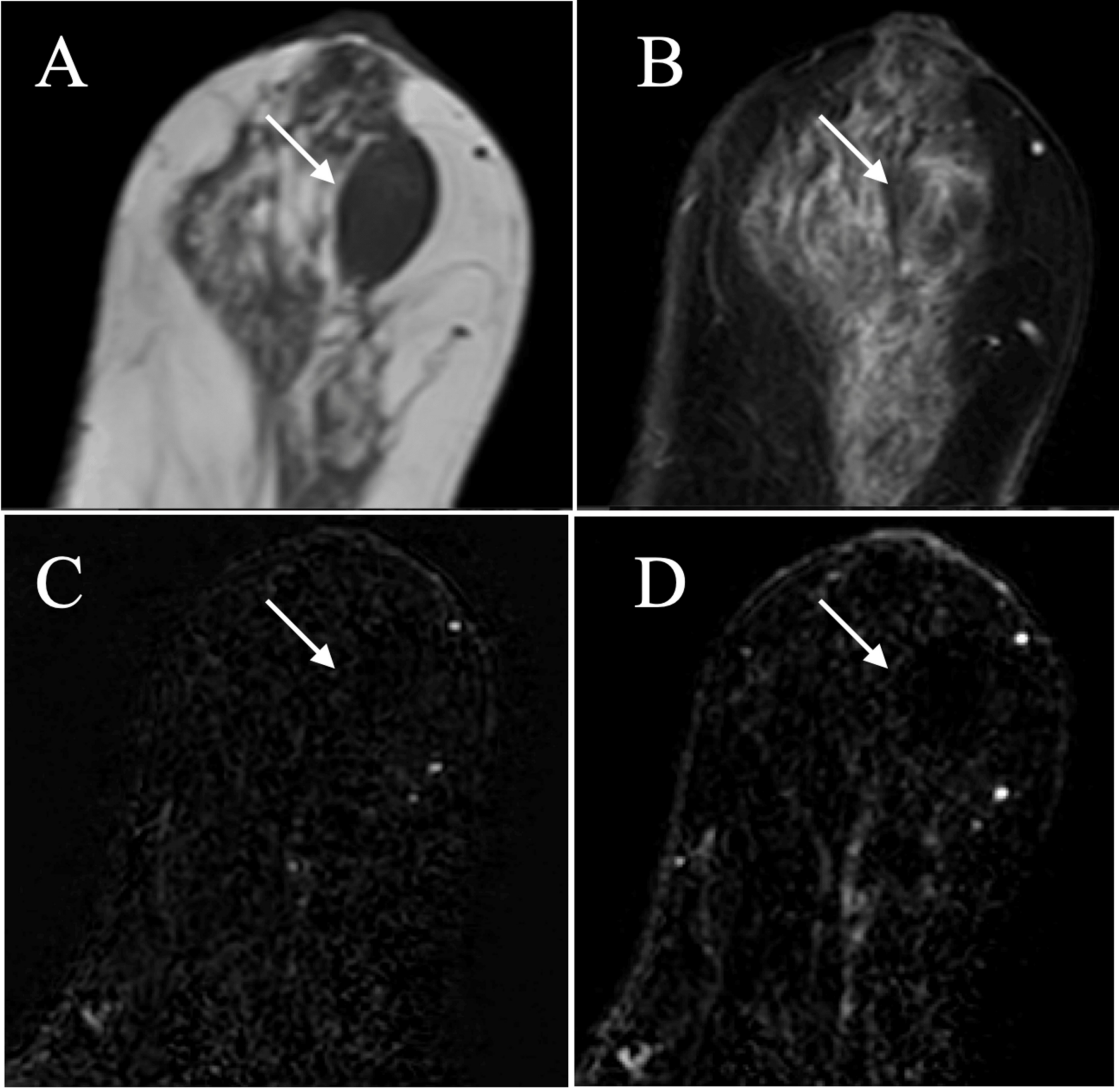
Magnetic resonance imaging (MRI) findings MRI of the breast mass (arrow) showed low signals on T1-weighted images (A), predominant low signals on fat-suppressed T2-weighted images (B), and no enhancement on dynamic studies (C and D).
